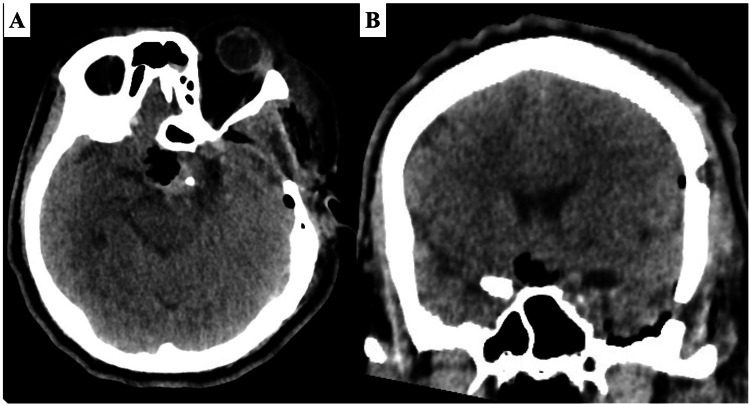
Post-operative non-contrast CT Axial (A) and coronal (B) images show a reassuring absence of any residual tumor.
